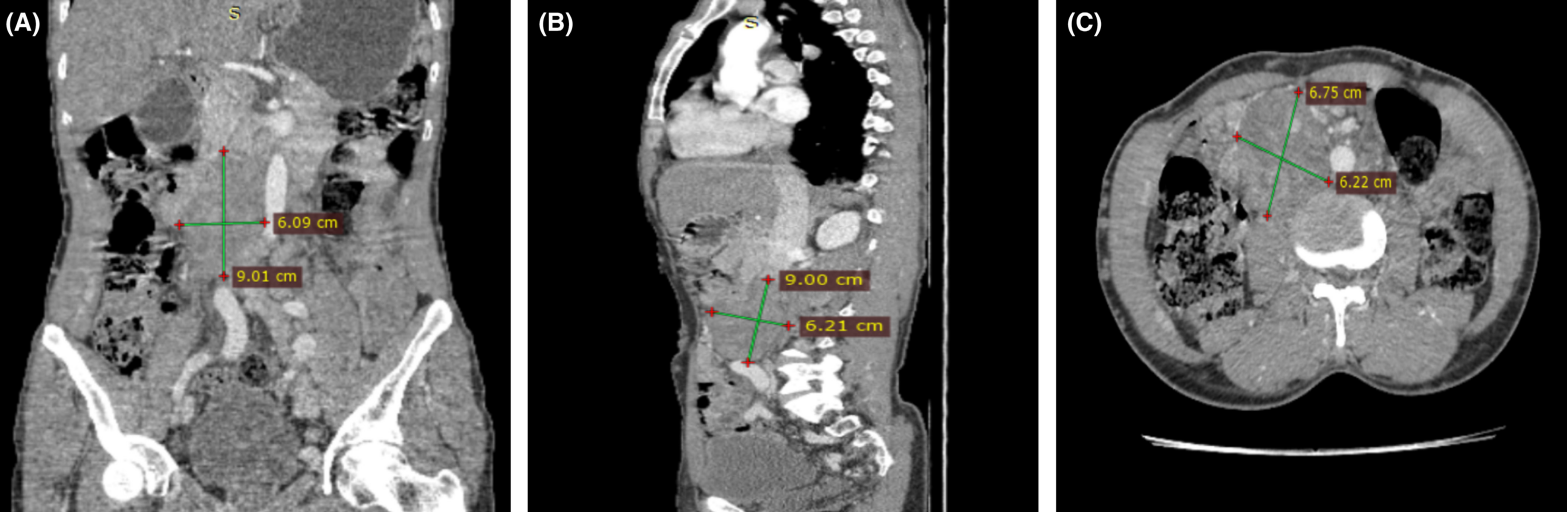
Preoperative Computed Tomography (CT) Angiogram of the Abdomen at images (A–C) (A) Coronal view showing a heterogeneously enhancing mass involving the whole length of the infrarenal inferior vena cava, (B) Sagittal View revealing a 9 × 6.2 cm inferior vena cava mass, (C) Axial View showing a mass obstructing the inferior vena cava lumen.
